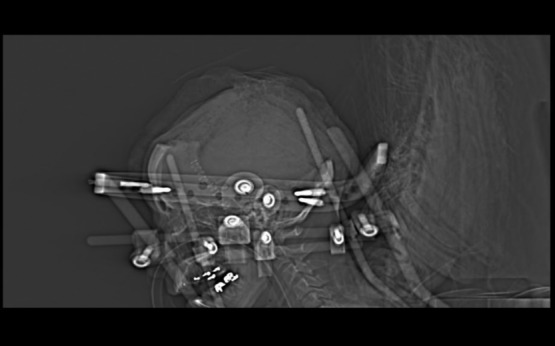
Sagittal computed tomography of patient's head showing the extent of skull debridement required for treatment.
